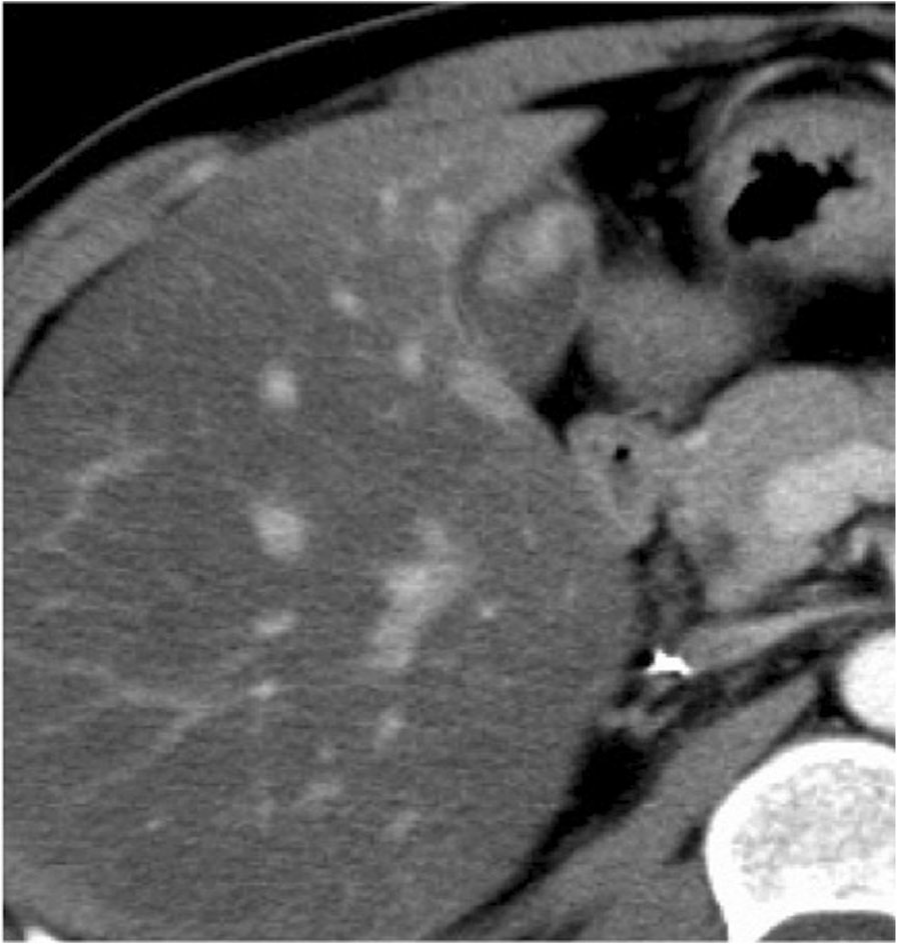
A contrast-enhanced CT scan showed a 20-mm lesion of the gallbladder with a contrast-enhanced effect
